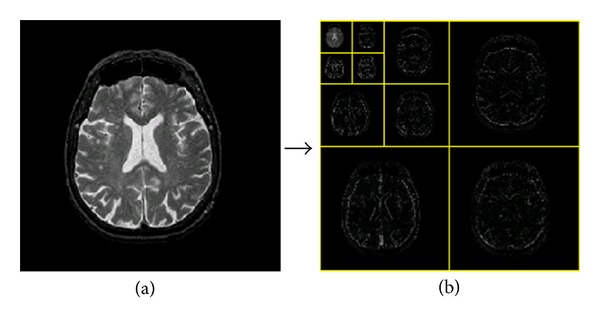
The procedures of 3-level 2D DWT: (a) normal brain MRI; (b) level 3 wavelet coefficients.
